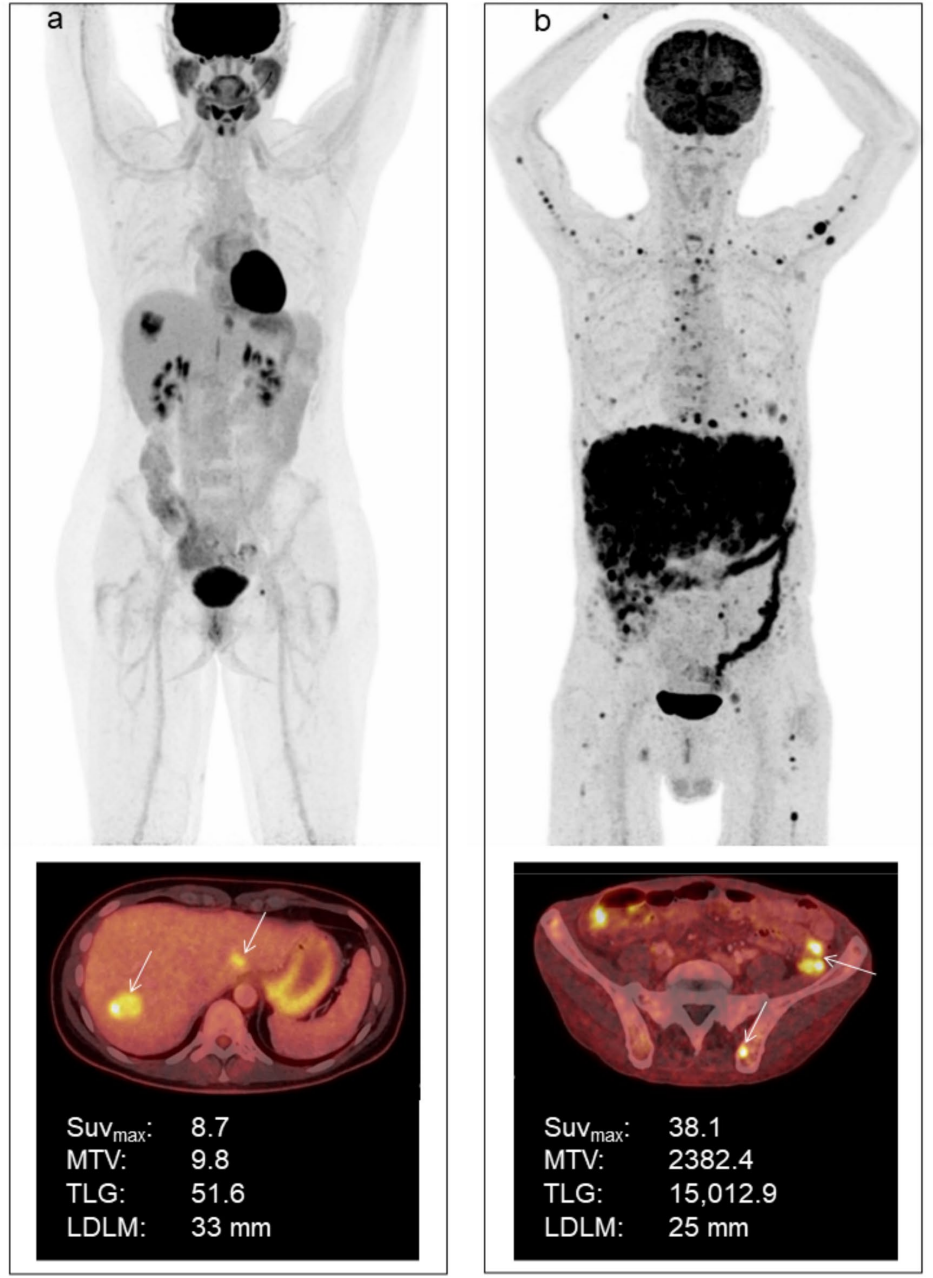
(a) Whole-body ^18^F-FDG PET/CT image of a female patient with two liver metastases from posterior uveal melanoma with an overall survival of 18 months. The two liver metastases are marked with arrows on the axial image; b) Whole-body ^18^F-FDG PET/CT image of a male patient with posterior uveal melanoma metastasized to various organs, including liver, lung, pleura, bone, muscle, lymph nodes, and the thyroid, and with an overall survival of 1 month. The axial image shows a metastasis in the right iliac bone and two carcinosis elements (arrows).
